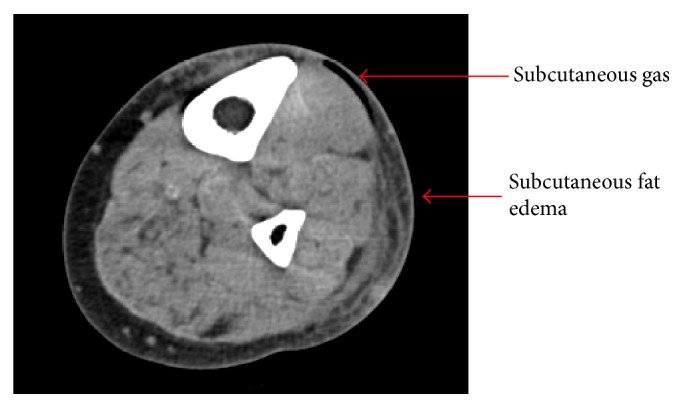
CT scan of the left lower extremity is displayed. Small amount of gas and subcutaneous fat edema present in anterior and lateral leg.
